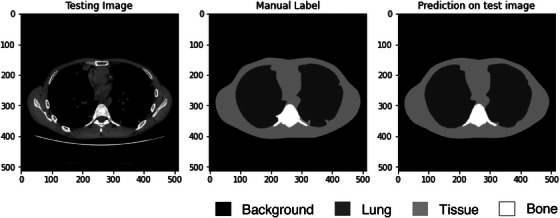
Results for automated segmentation using the U−Net model of the three classes of tissues and the background region shown in transaxial thoracic computed tomography images.
